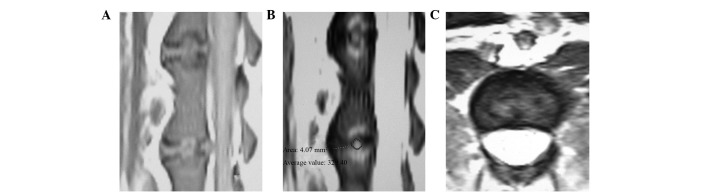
Images at 24 weeks after injection of 0.3 ml polymethylmethacrylate (A) T1-weighted image shows irregular and patchy low signals in the intervertebral space, where the intervertebral disc exhibited an abnormal shape. (B) T2-weighted images show that the area of relatively high signal declined. (C) Axial T2-weighted image shows the irregular shape of the nucleus pulposus, and the patchy high signals in the annulus fibrosus, which did not have a clear boundary with the nucleus pulposus.
